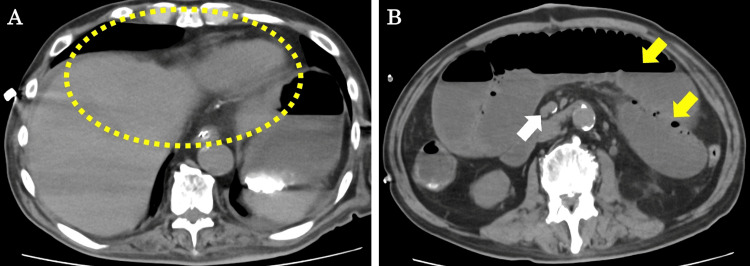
CT findings on POD 6 (A) PVG has disappeared (yellow dotted lines). (B) Superior mesenteric venous gas (white arrow) and PI have disappeared (yellow arrows). CT, computed tomography; POD, postoperative day; PVG, portal venous gas; PI, pneumatosis intestinalis
